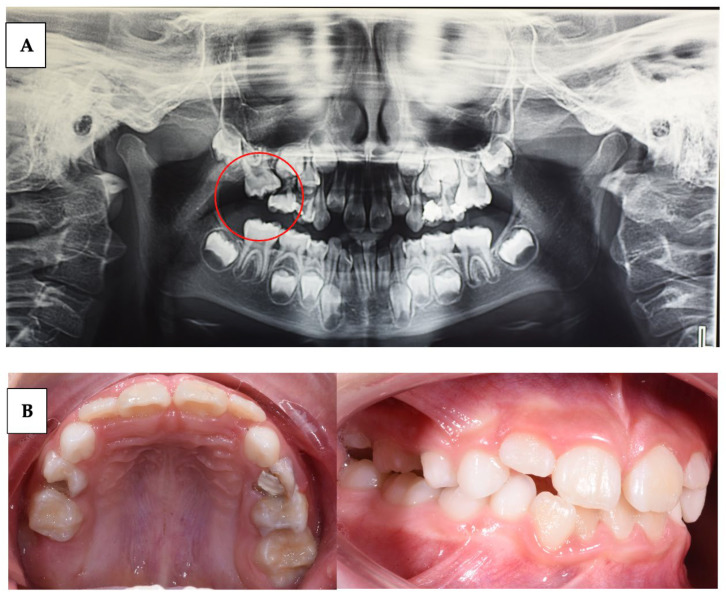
(A) Panoramic radiograph of a suspected case of PFE on tooth 16; (B) intraoral aspect of suspected PFE on tooth 16.
